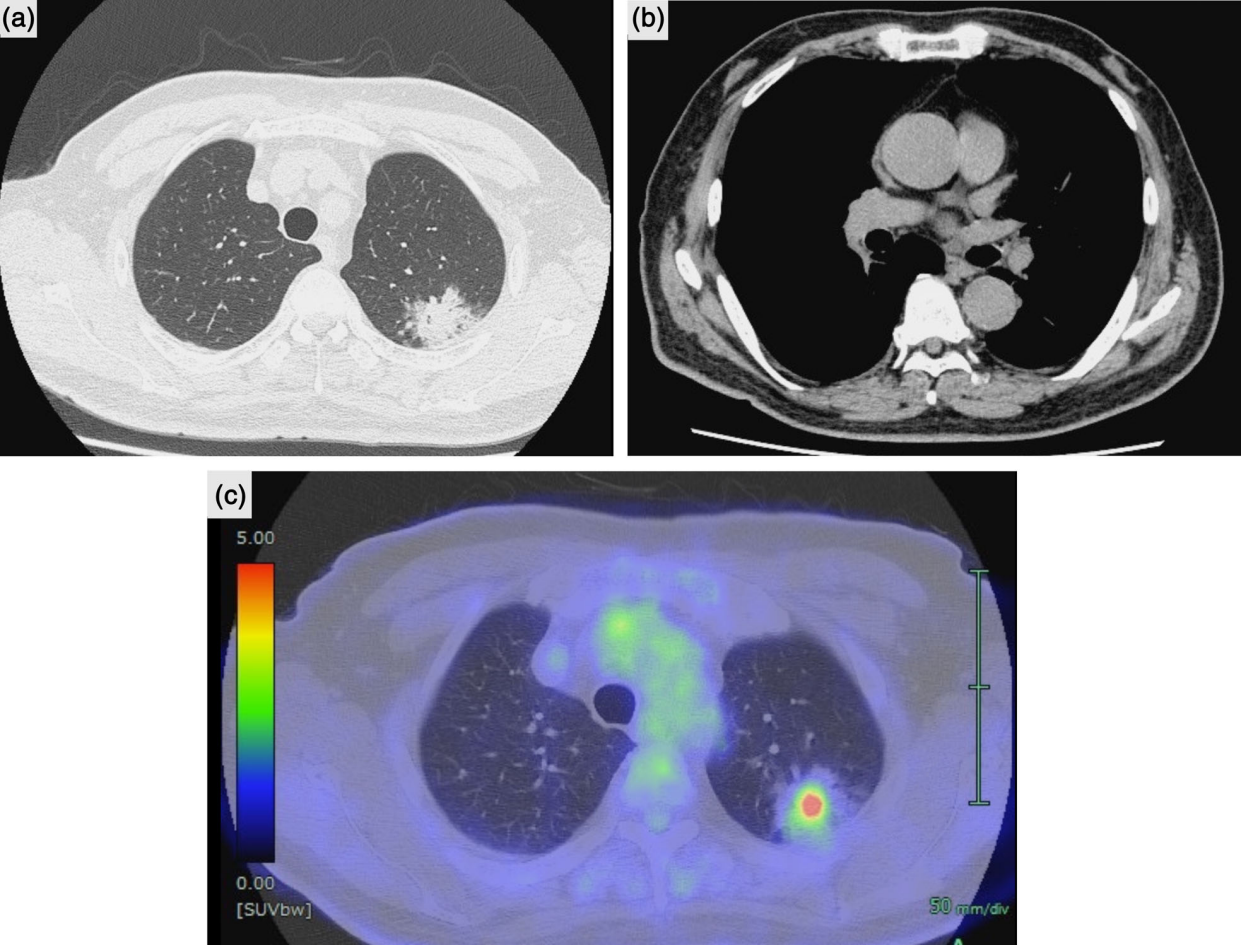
(a, b) Preoperative chest computed tomography. Computed tomography shows a 43‐mm nodule at the periphery of S^1+2^ of the left upper lobe. There is no obvious lymph node enlargement. (c) The maximum standard uptake value of the positron emission tomography is 7.5.
